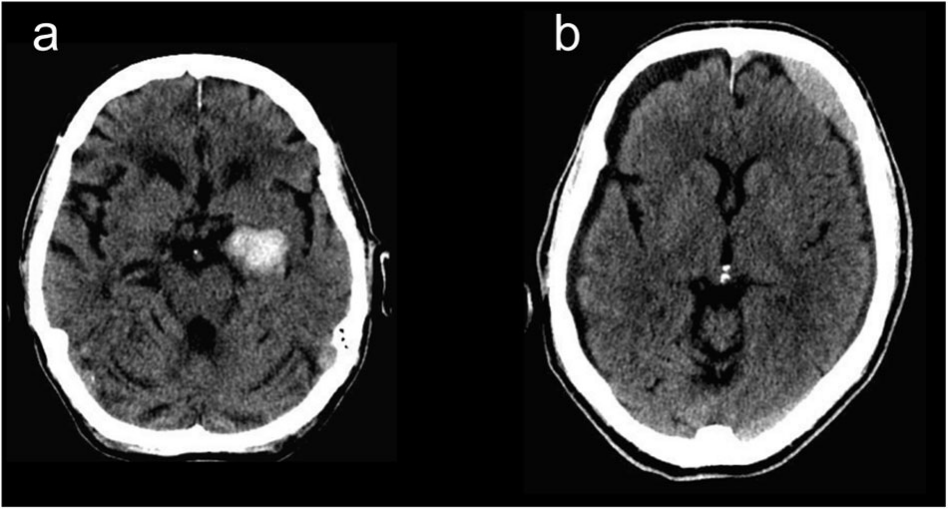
Representative head CT image slices associated with case #1 (a) and case #2 (b)
